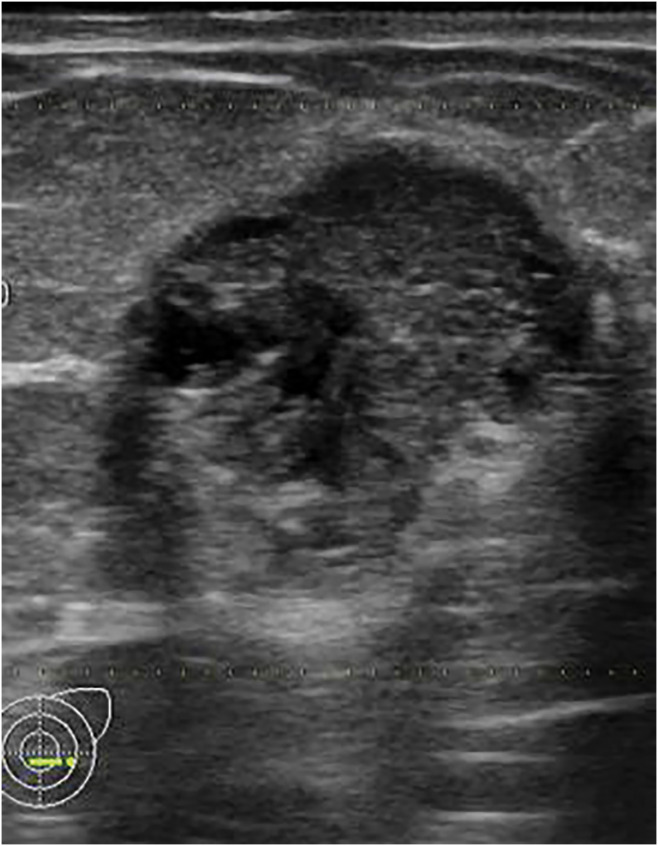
Ultrasonography showing a circumscribed isoechoic tumor measuring 26 mm with enhanced posterior echo.
